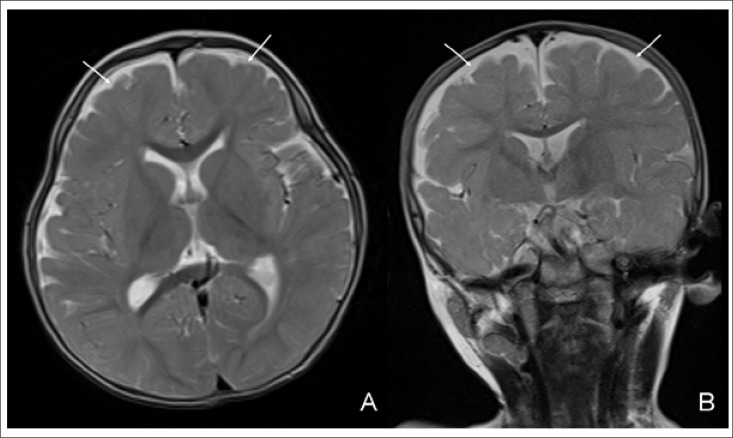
Control MR images after therapy. Axial (A) and coronal (B) T2-weighted images demonstrated recovery of cerebral atrophy. Subarachnoid space width was in normal range (arrows)
